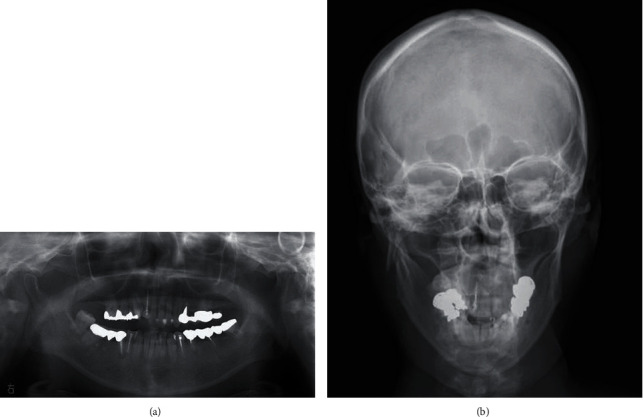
(a) Panoramic X-ray at the first visit. A right mandibular condylar base fracture is observed. (b) A simple head X-ray at the first visit (P-A). A fracture of the right mandibular condyle and external displacement of the bone fragment are observed.
